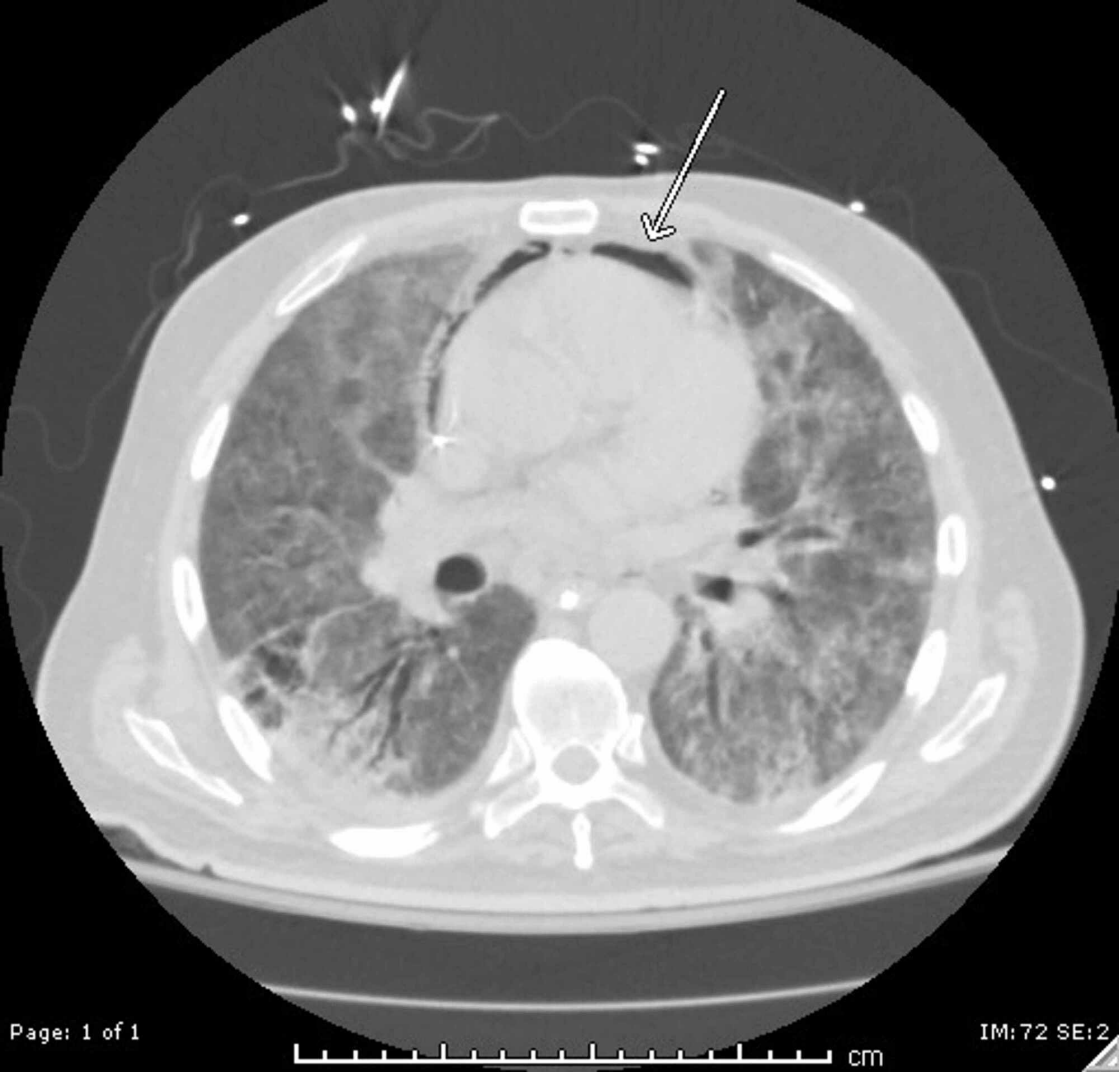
A lung window CT image of an inferior portion of the thorax showing pneumopericardium (arrow).
